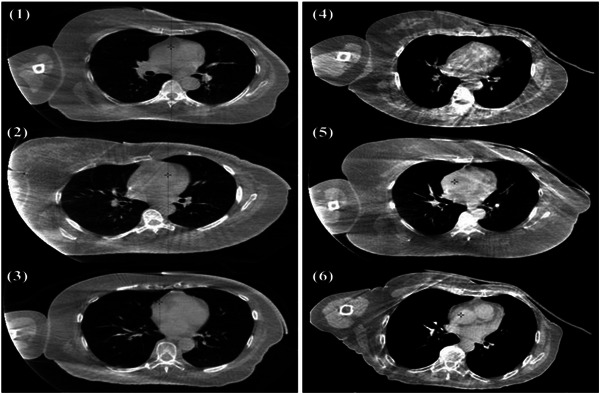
Cone‐beam computed tomography (CBCT) images of patients using conventional bonus (CB) and 3D‐printed bolus (3DPB) ((1–3): 3DPB, (4–6): CB).
